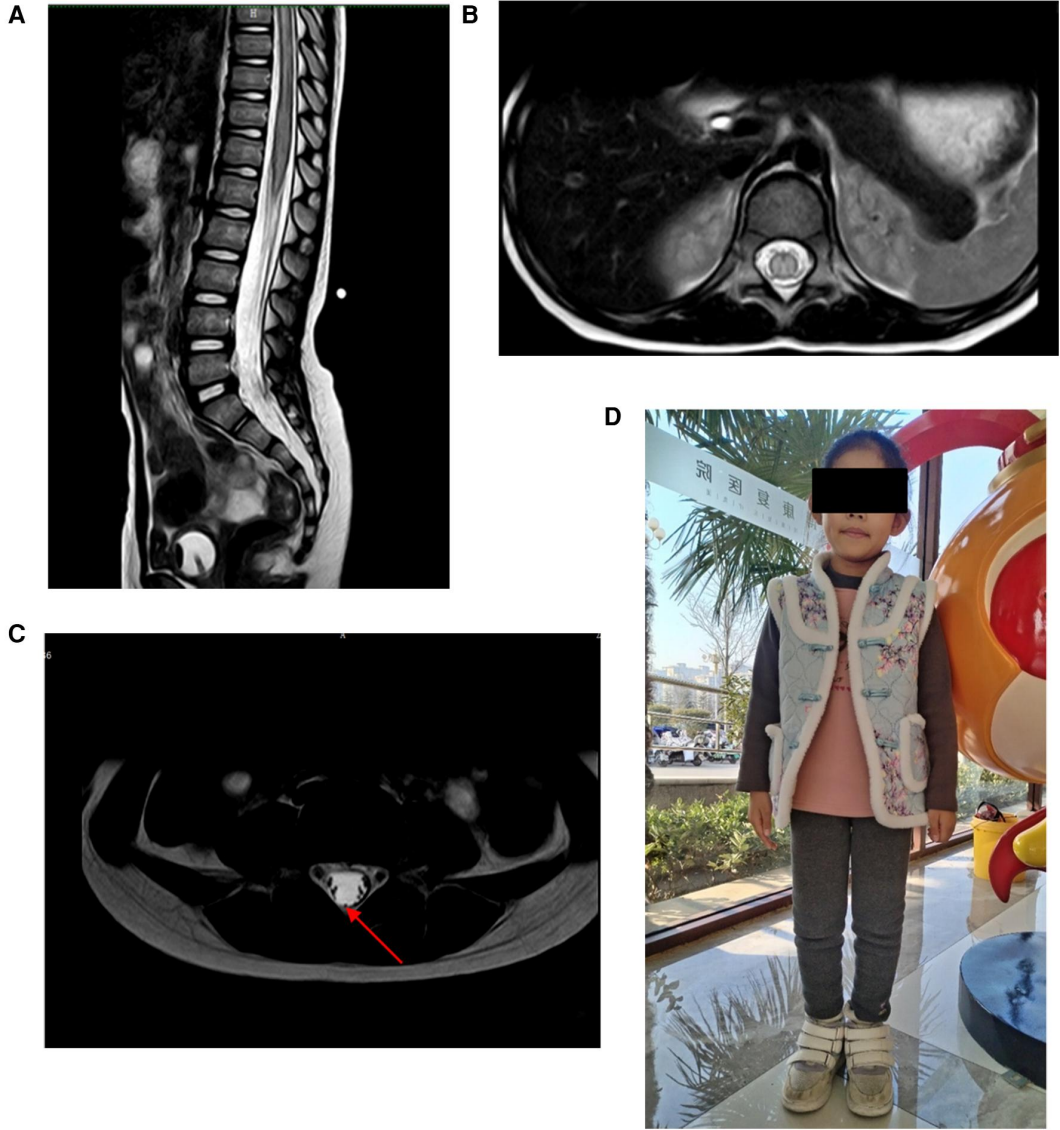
(A,B) MRI revealed spinal cord edema. (C) MRI revealed thickening of the filum terminale (red arrow). (D) At 6 months follow-up, the patient could walk with assistive devices.
